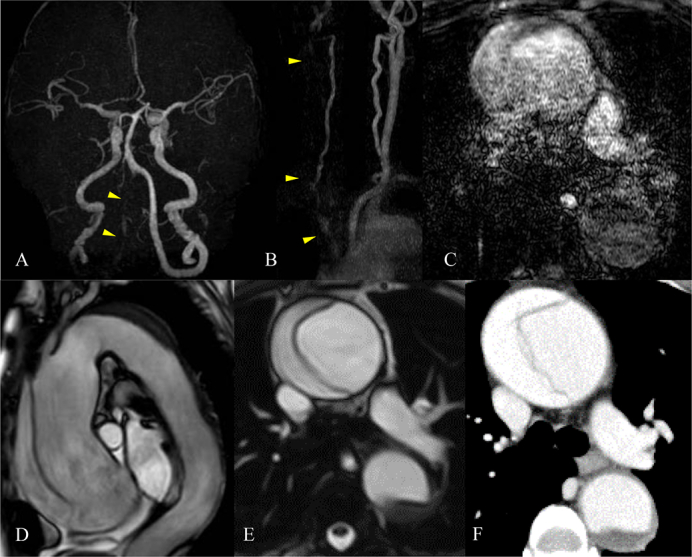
CT and MR imaging of the brain, neck, and thoracic aorta. The right distal VA in the brain MRA (yellow allow) and the right ICA show a slightly lower signal intensity than the left ICA (A). The brachiocephalic artery in the cervical MRA is invisible (yellow arrow), and the right vertebral artery can be partially observed (B). The oblique sagittal pulse-gated noncontrast thoracic bSSFP-MRA image clearly shows a dissection flap in the dilated ascending aorta (D). Comparing TOF-MRA (C), pulse-gated noncontrast thoracic bSSFP-MRA (E), and chest CTA (F), pulse-gated noncontrast thoracic bSSFP-MRA produced a better imaging quality for flap detection. CT, computed tomography; MR, magnetic resonance; VA, vertebral artery; ICA, internal carotid artery; MRA, magnetic resonance angiography; bSSFP, balanced steady-state free precession; TOF, time-of-flight; CTA, computed tomographic angiography. (For interpretation of the references to colour in this figure legend, the reader is referred to the web version of this article.)
